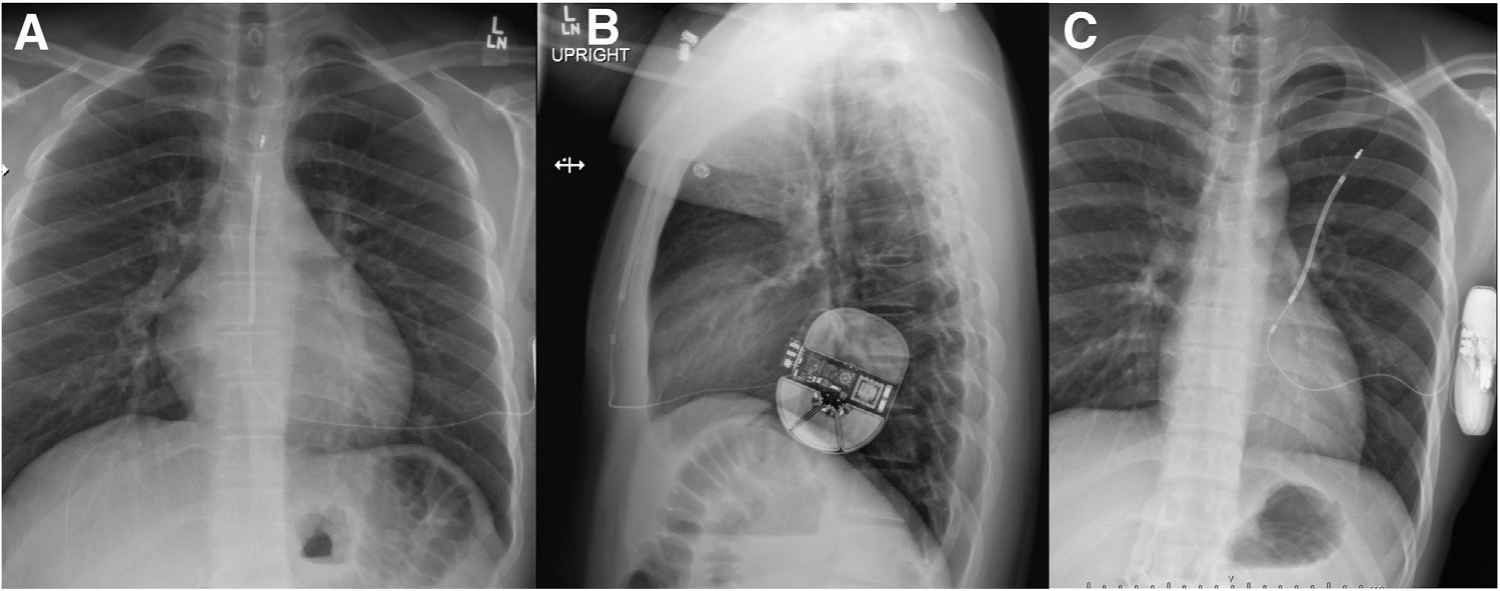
Anteroposterior and lateral chest radiographs. A: The conductor coil of the subcutaneous implantable cardioverter-defibrillator (SICD) is subcutaneously tunneled from the anterior chest wall to the xiphoid process. B: The conductor is then subcutaneously tunneled to the left axilla, where the generator is placed. C: This patient did not achieve appropriate SICD sensing and defibrillation on initial device placement because the coil and generator did not vector across the majority of ventricular myocardium.
